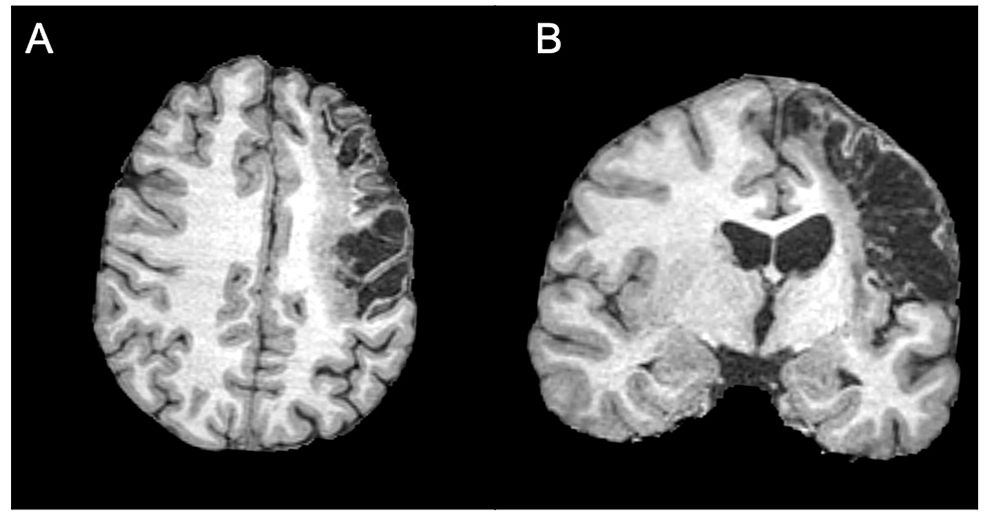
T1-weighted MRI, axial (A) and coronal (B) views, demonstrates extensive cystic encephalomalacia and gliosis in the territory of the cortical branches of the superior division of the left MCA. MCA: middle cerebral artery.
